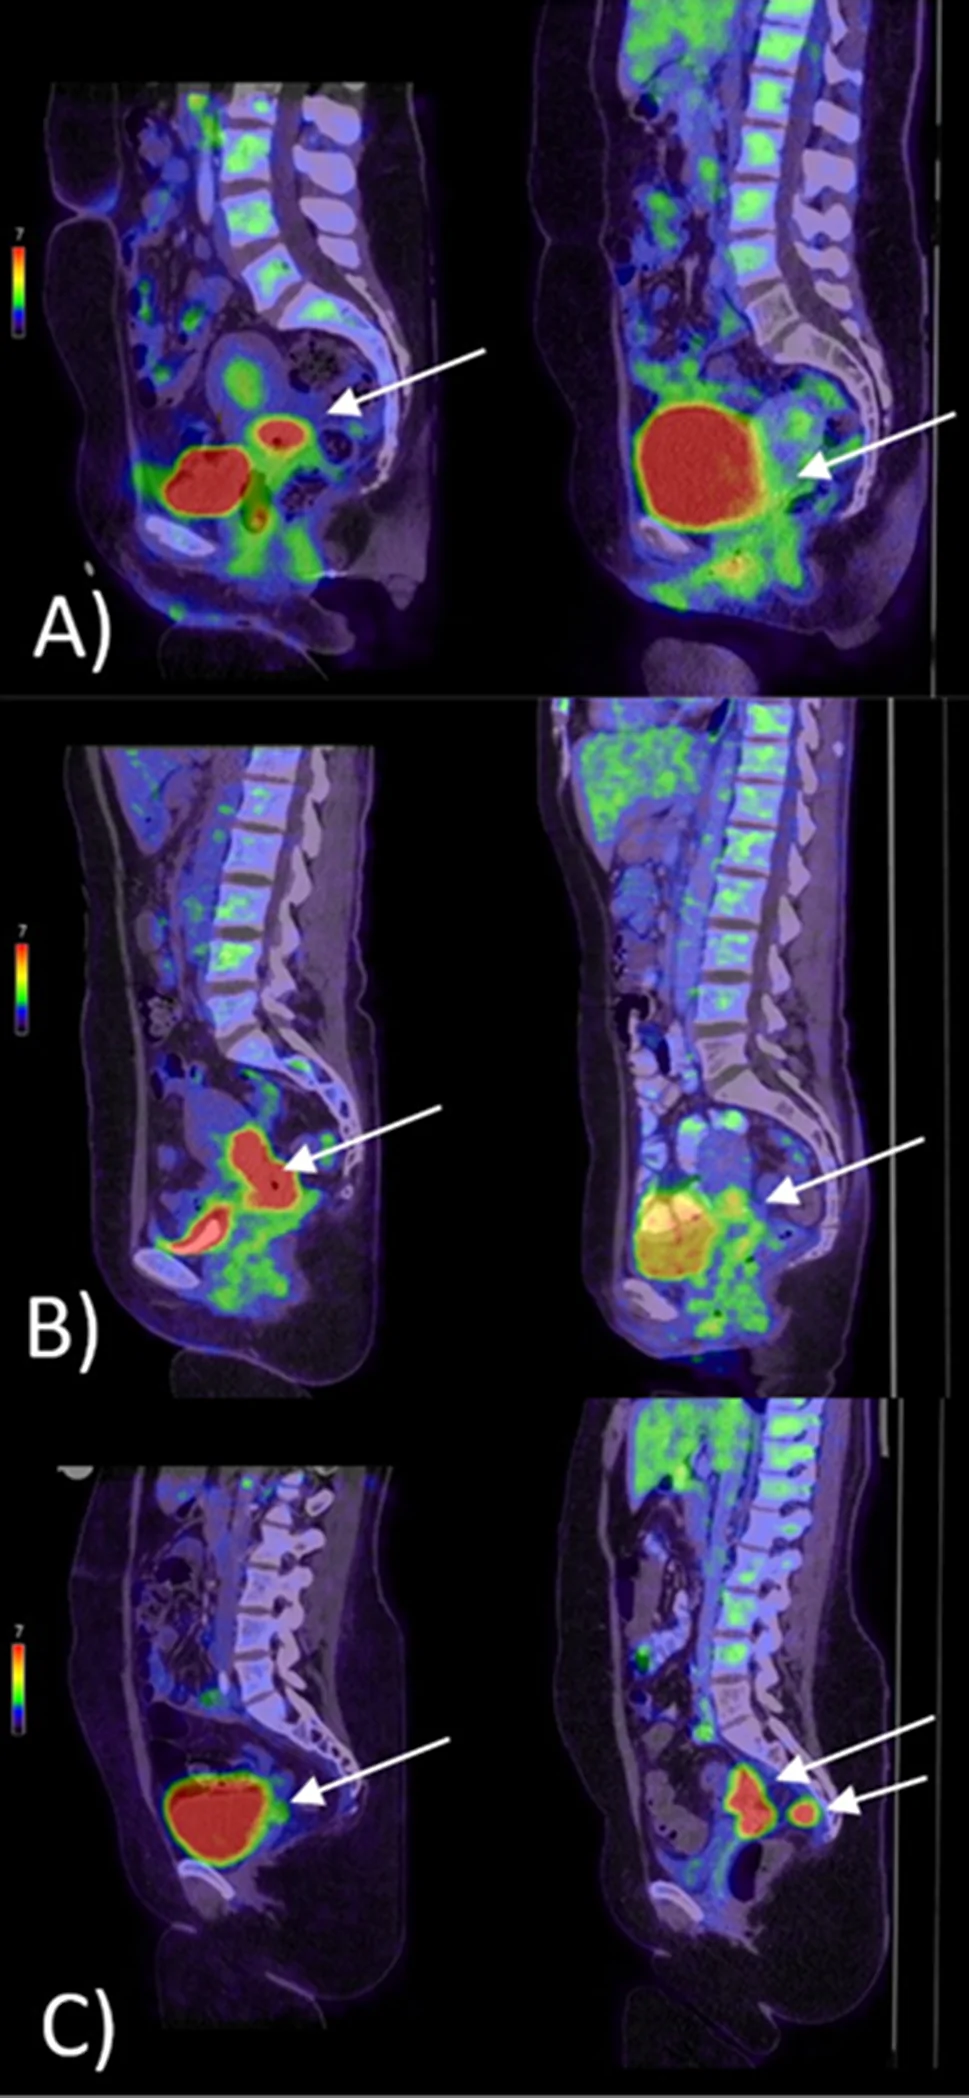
Fused PET-CT images of patient with (A) CMR (B) PMR and (C) PMD (as measured with any of the PET-parameters). Pre-treatment to the left and post-treatment to the right. White arrow indicates tumour (and metastasis in case C)) localisation. CMR: complete metabolic response, PMR: partial metabolic regression, PMD: progressive metabolic disease
